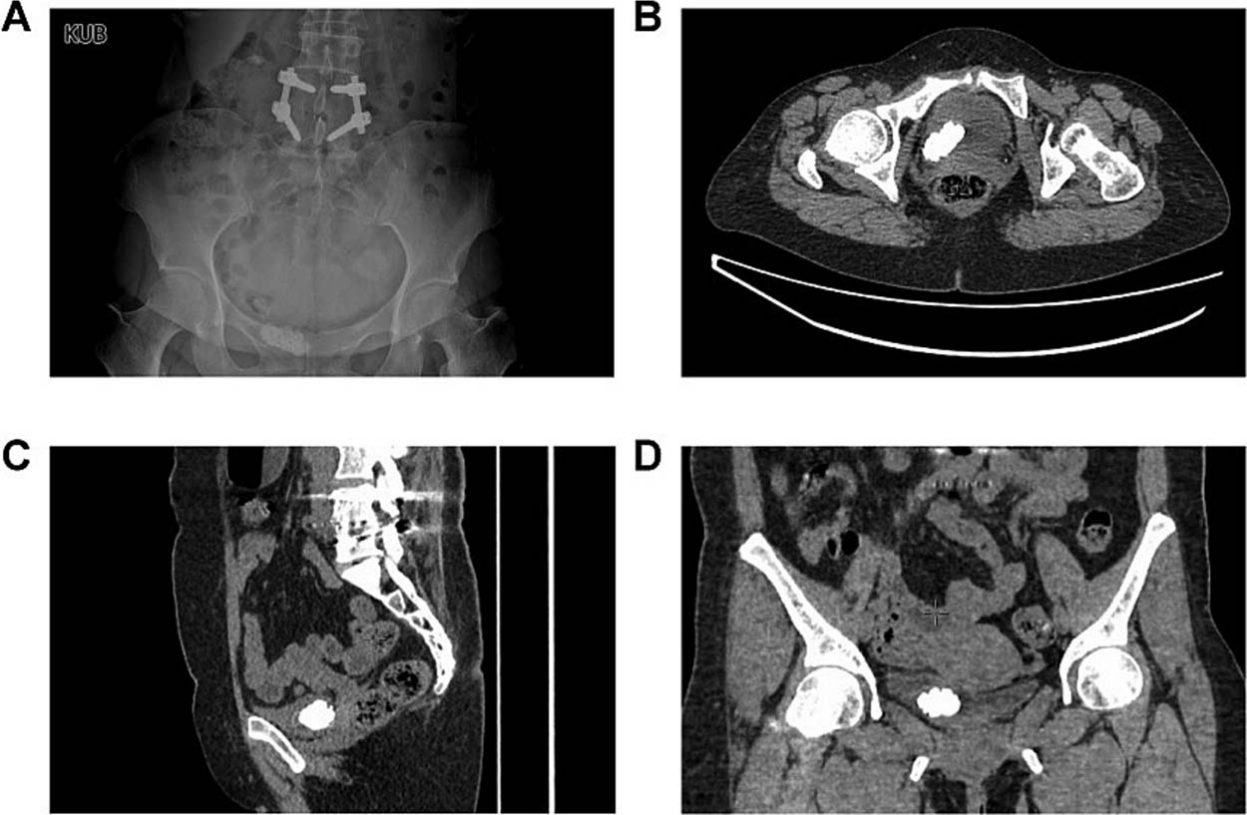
The preoperative imaging examinations of the patient. (A) The plain film of kidney–ureter–bladder. (B–D) Computed tomography.
